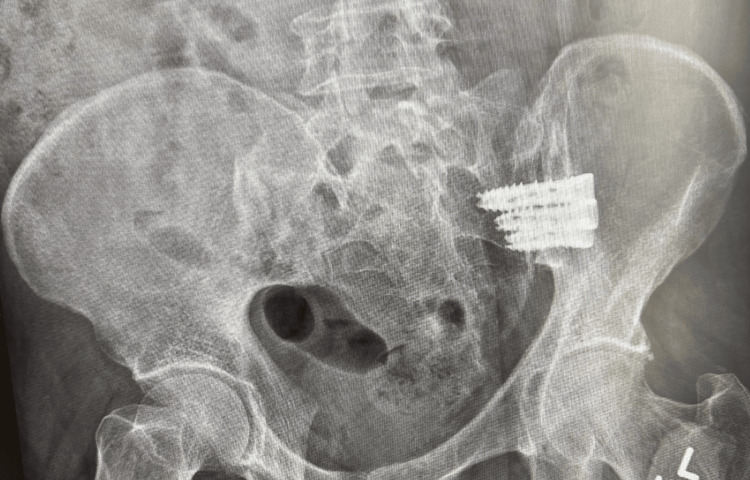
Postoperative Pelvic Inlet Radiograph Six-month follow-up imaging demonstrating maintained structural integrity of the implants and successful alignment within the ilium and sacrum.
